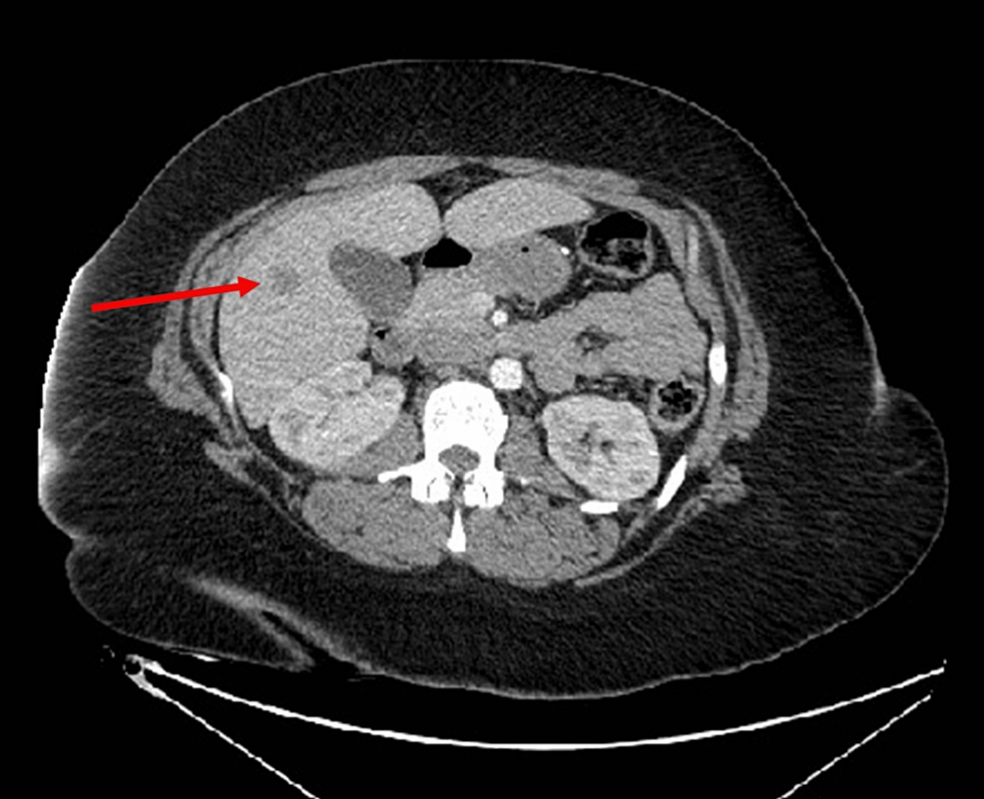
CT abdomen and pelvis with contrast A 1.5 cm hypodensity in segment 6 of the right lobe of the liver (arrow), which raises suspicion for metastatic disease in setting of malignancy.
